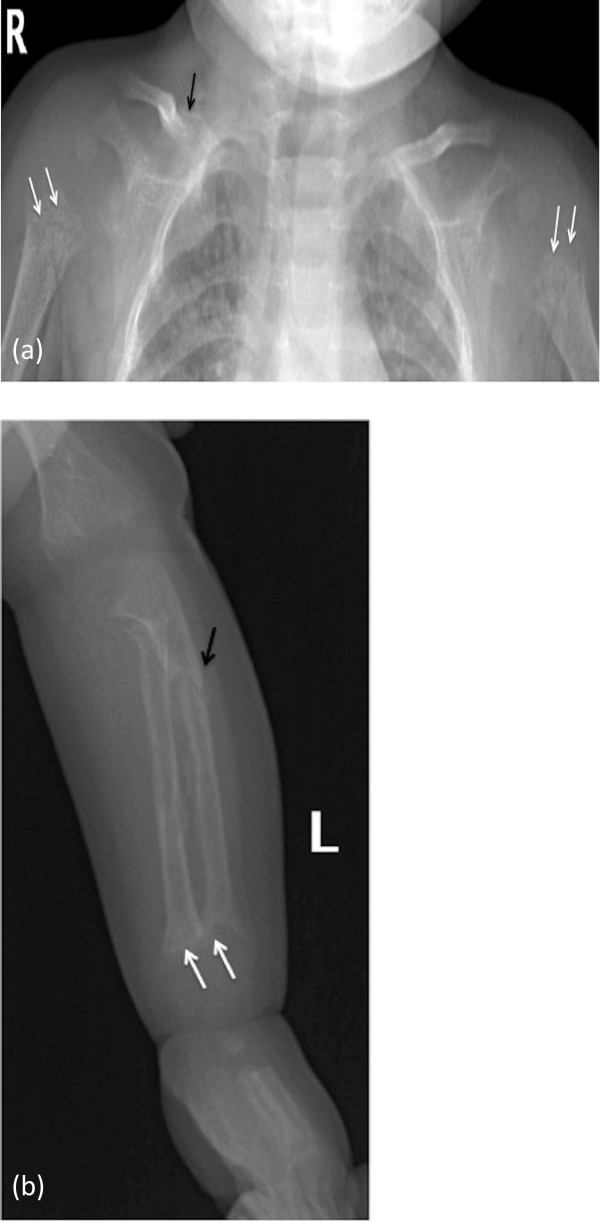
X-ray images at time of presentation showing generalized osteopenia with altered texture (a & b). Humeral metaphysis shows frying with more pronounced lucency (a), and ulnar/radial metaphysis shows frying and cupping of their outline (b) - (white arrows). The cortices are indistinct with coarse fuzzy trabecuale (a & b). Fracture of right clavicle (a), and proximal shaft of the ulna (b) - (black arrows).
